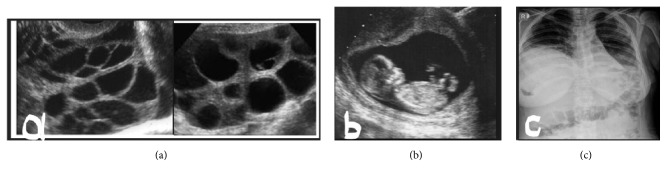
(a) Multicystic appearance in both ovaries (left ovary, 14*∗*13 cm; right ovary, 13*∗*12 cm). (b) A single intrauterine pregnancy with gestational age of 12 weeks according to the crown-rump length. (c) On chest X-ray, the costophrenic angles were closed with appearance of hydrothorax.
